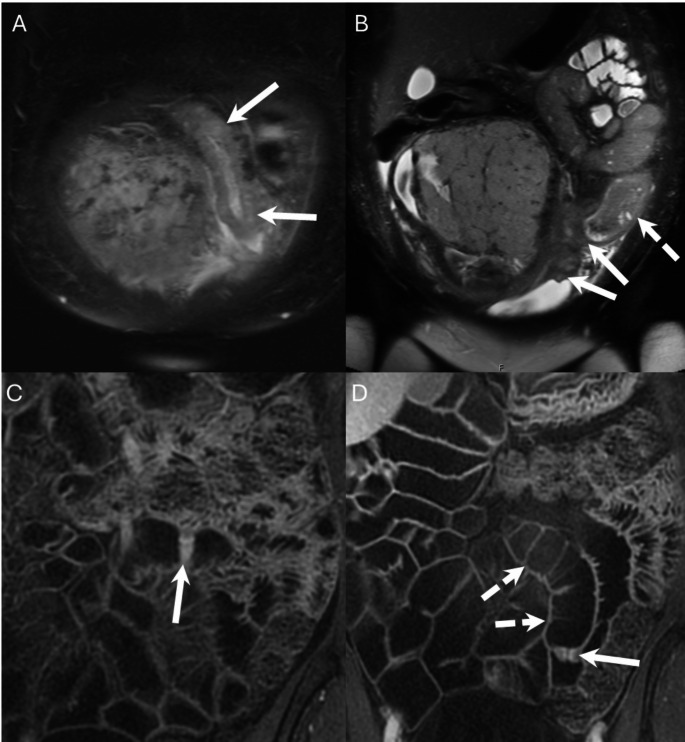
A, B 23-year-old female pregnant at 22 weeks 1 day. Patient presents with progressively worsening generalized abdominal pain for 2 months, anemia and rectal bleeding previously ascribed to hemorrhoids. Non contrast MRI was obtained primarily to assess for causes of abdominal pain. (A, B) Coronal T2 fat saturated images show marked thickening and edema of a long segment of the distal ileum (arrows, A), a fistula between the ileum and urinary bladder (arrows, B) and pre-stenotic bowel dilation (dashed arrow, B). C, D 64-year-old female with chronic low back pain and anemia treated with iron supplementation. Coronal post gadolinium images demonstrate several diaphragm type strictures, two of which are shown (arrows, C and D), associated with mild dilation of the upstream bowel (dashed arrows, D). The findings are consistent with diaphragm disease and the patient was found to be taking high doses of NSAID medications
